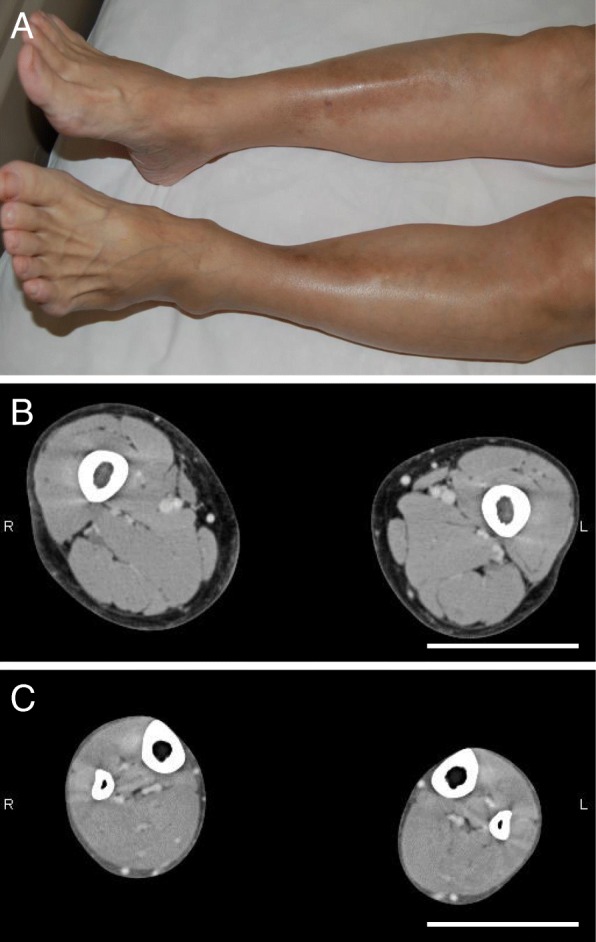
Loss of subdermal adipose tissues in the lower legs in Case 2. a She manifested the loss of subdermal adipose tissues of the lower legs. b The subdermal adipose tissues of the thighs were spared from lipoatrophy, as revealed by MRI. c The subdermal adipose tissues of the lower legs were almost completely lost in the lower legs, as revealed by MRI
